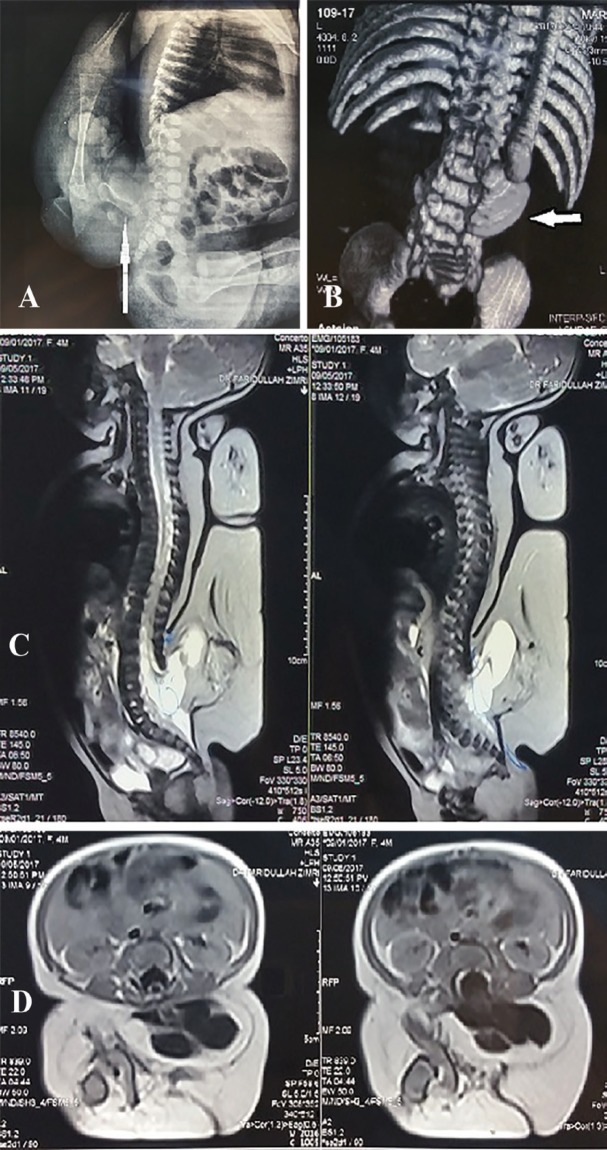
A: Plain X-rays showed the osseous components of the accessory lower limb. The arrow is pointing towards the rudimentary hemi-pelvis. B: Lumbosacral computed tomography with three dimensional reconstruction revealed deficient posterior bony elements at the level of L2 through SI vertebrae along with evidence of lipomyelomeningocele. It also confirmed an accessory lower limb attached to the right gluteal muscles. The limb had a rudimentary iliac bone (the arrow is pointing towards it), femur, tibia, fibula, rudimentary foot bones, hip and knee joints. C: MRI lumbosacral spine, sagittal view. D: MRI lumbosacral spine, axial view. The whole spine Magnetic resonance imaging (MRI) confirmed the presence of spinal dysraphism involving lumbar spine with deficient posterior elements. The spinal cord and thecal sac were protruding posteriorly and towards the left superiorly through the defect in the posterior elements at the level opposite L3 through S1 vertebrae, revealing a large lipomyelomeningocele measuring approximately 4.7×3×4.8 cm. The CT scan findings of the accessory lower limb were re-confirmed
